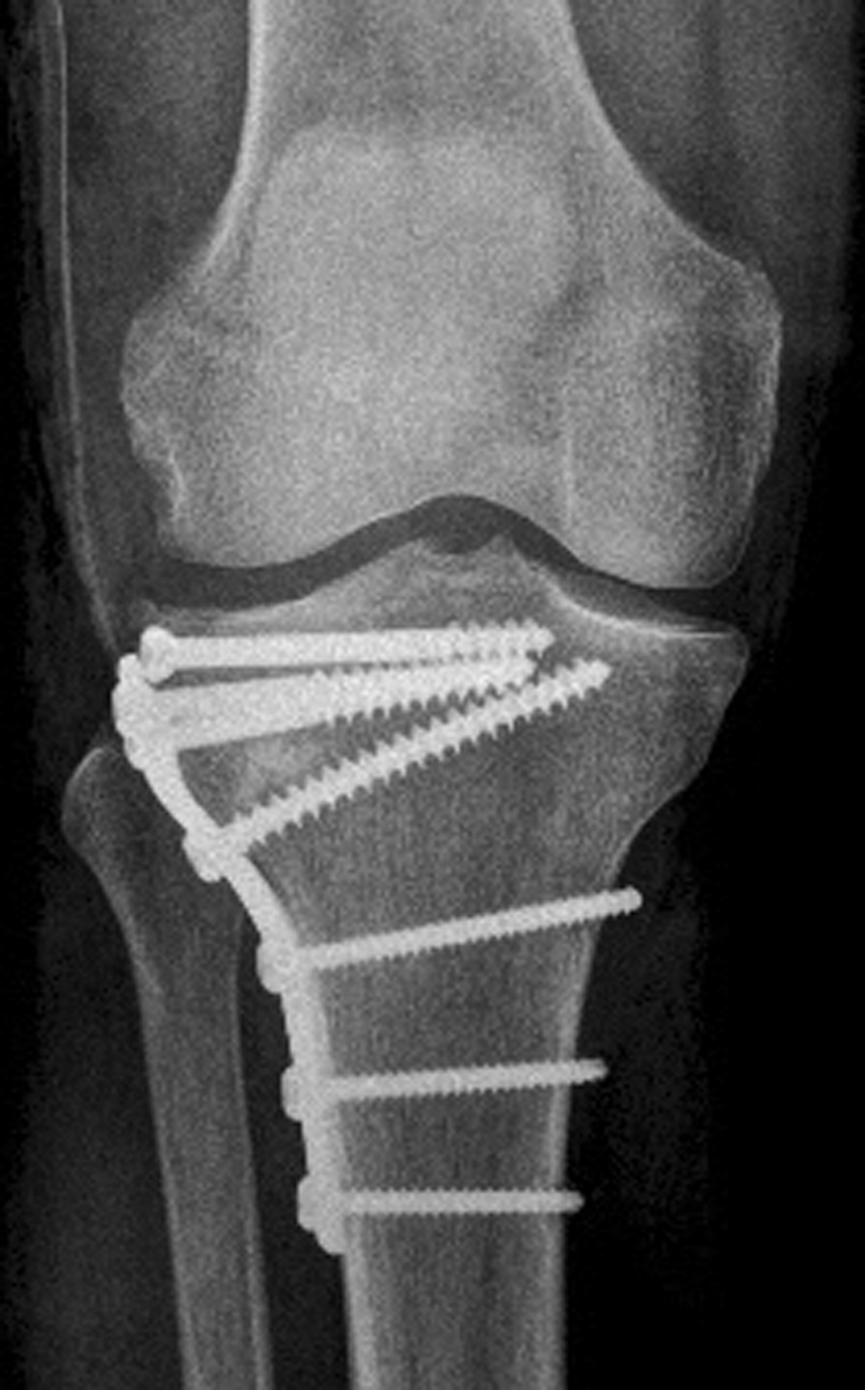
Postoperative anteroposterior (a-p) X-ray of the knee showing the result of reconstruction. The subchondral bone defect was filled with CBVF.
